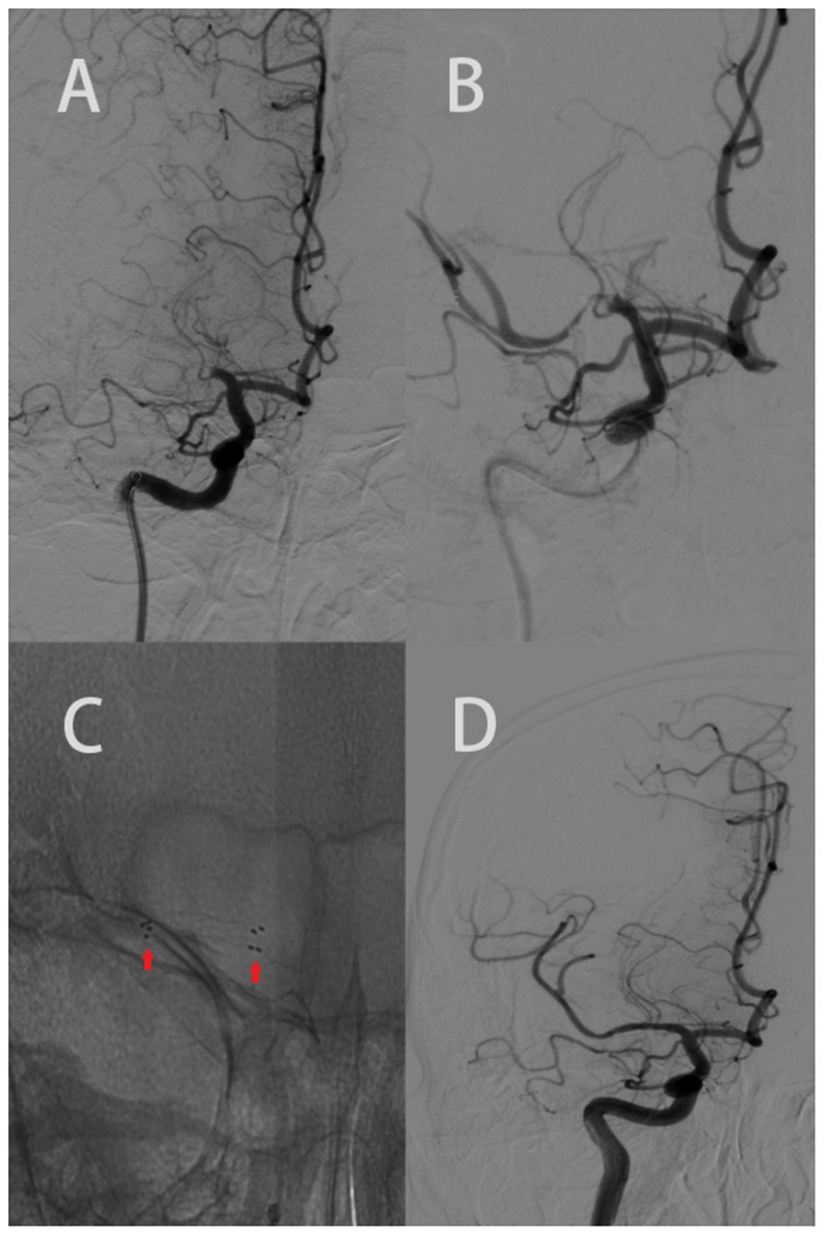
Elderly patient with an acute onset of left body weakness, numbness, and difficulty speaking (NIHSS 7). The ASPECTS was 9 based on CT scan. (A) AP right carotid angiogram clearly showed an occlusion of the M1 segment of the right MCA. (B) Revealed the post failed MT with stenosis. (C) The red arrow shows the proximal and distal legs of the stent. (D) AP right carotid angiogram depicting recanalization of the right MCA and its branches (mTICI 3).
